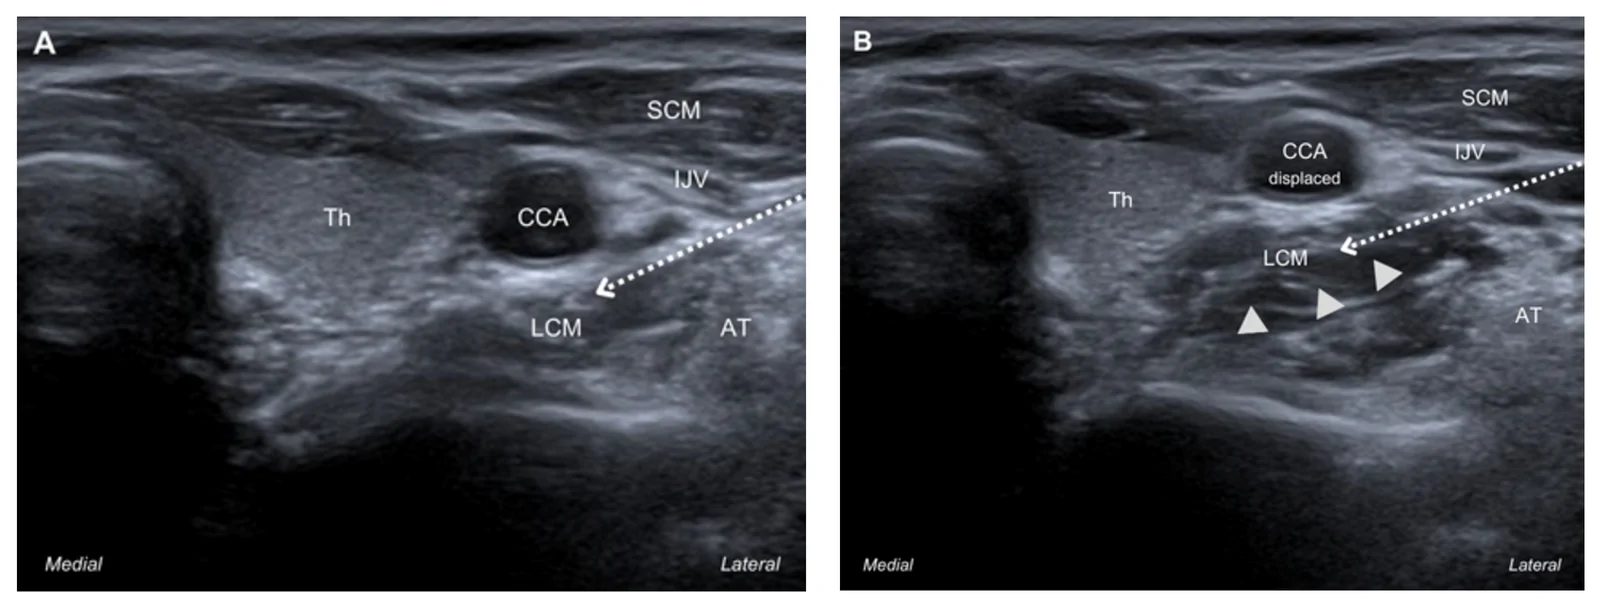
Ultrasound-guided stellate ganglion block technique. (A) Baseline axial ultrasound image at the C6 level showing the relationship between the prevertebral structures. The common carotid artery (CCA) is shown in a neutral position, partially obscuring the lateral aspect of the longus colli muscle (LCM). The dashed arrow indicates the intended in-plane needle trajectory toward the prevertebral fascia, directly anterior to the LCM. (B) Intra-injection image showing local anesthetic spread beneath the prevertebral fascia (white arrowheads), with secondary ventral and slight medial displacement of the common carotid artery (labeled ‘CCA displaced’), consistent with subfascial drug deposition and compartmental expansion. Abbreviations: SCM, sternocleidomastoid muscle; IJV, internal jugular vein; CCA, common carotid artery; LCM, longus colli muscle; AT, anterior tubercle of C6; Th, thyroid gland. Image orientation is indicated as medial and lateral.
